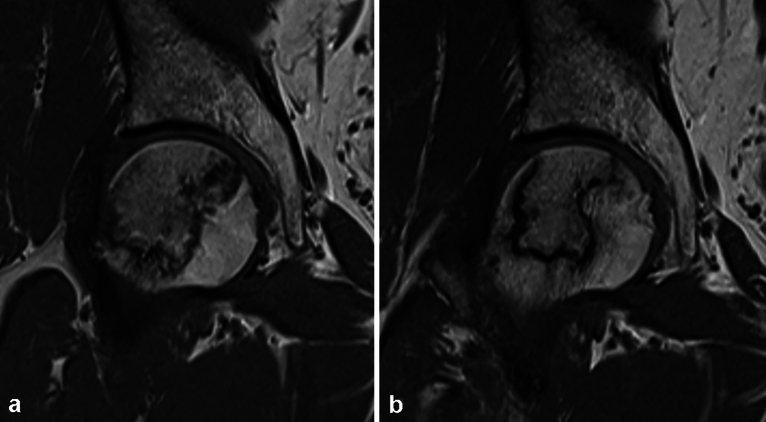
(a) MRI images showing a large necrotic lesion in the femoral head of a 44-year-old male patient. The calculated volume of the necrotic lesion was 23.77 cm^3^. (b) MRI image showing a decrease in volume of necrotic lesion 1 year after denosumab treatment. The calculated volume of the necrotic lesion was 14.33 cm^3^.
